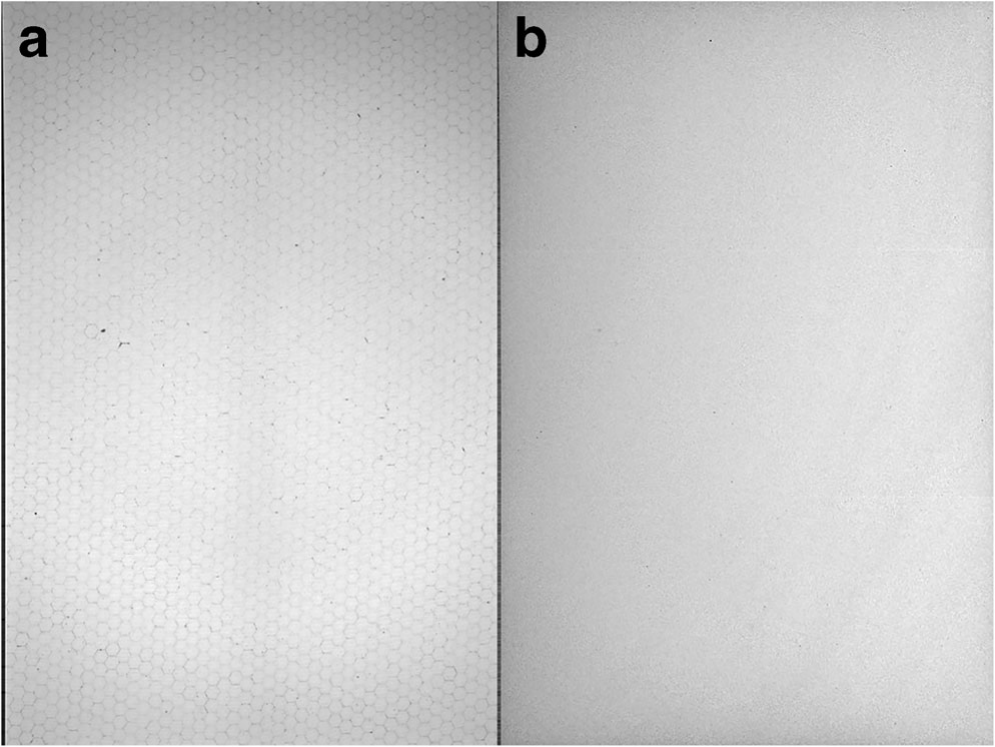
a A non-normalized and b normalized (*right*) CT projection image taken with no object in the CT FOV using parameters similar to those used by the facility for their specific imaging protocols.
